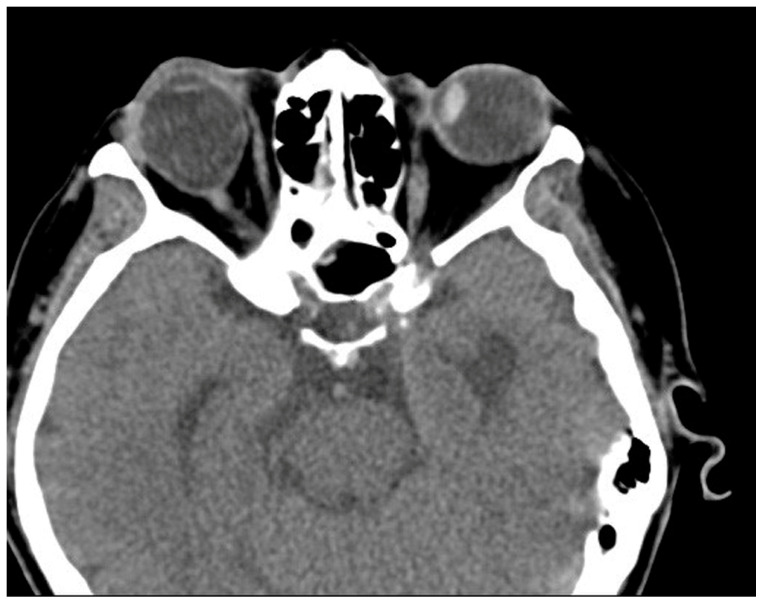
Preoperative CT image of Case 5. Thickening of the left medial rectus muscle with severe left esotropia is noted.
